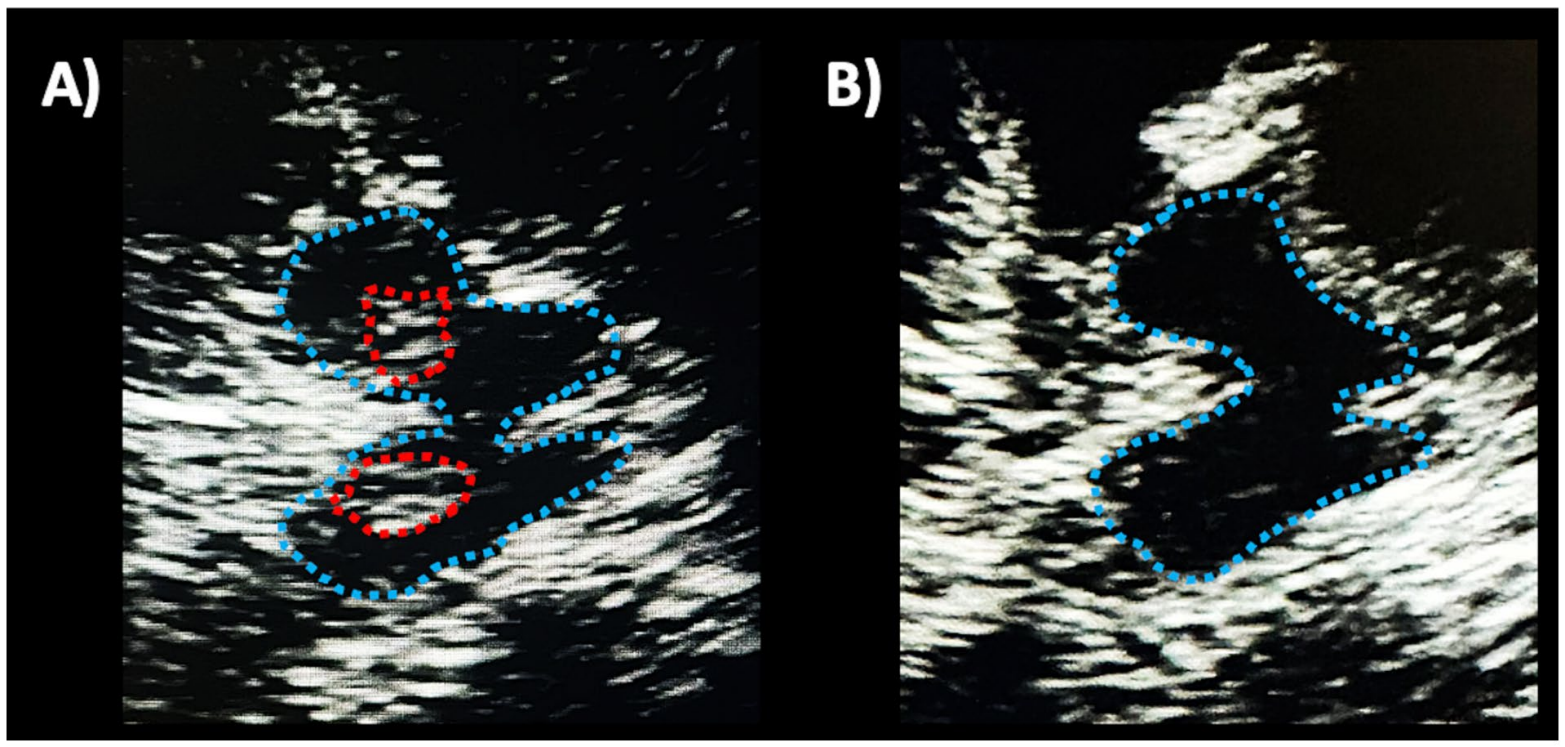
Transcranial sonographic images showing an axial view of the midbrain in a patient with Parkinson’s disease (A) and in a healthy subject (B). In both images, the midbrain is delimited by a light blue dotted line. In the left image, hyperechogenic (bright) areas are visible bilaterally within the substantia nigra, encircled by red dotted lines. On the contrary, the midbrain is homogenously dark in the right image, reflecting normal echogenicity.
